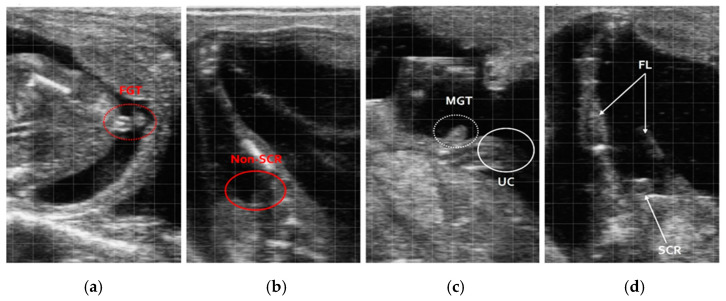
Still image ultrasound of a fetus between 86 and 100 days of pregnancy. (a) Female aerial view; (b) female posterior view; (c) male aerial view; (d) male posterior view; fore limbs (FLs); umbilical cord (UC); male genital tubercle (MGT); female genital tubercle (FGT); scrotum (SCR).
